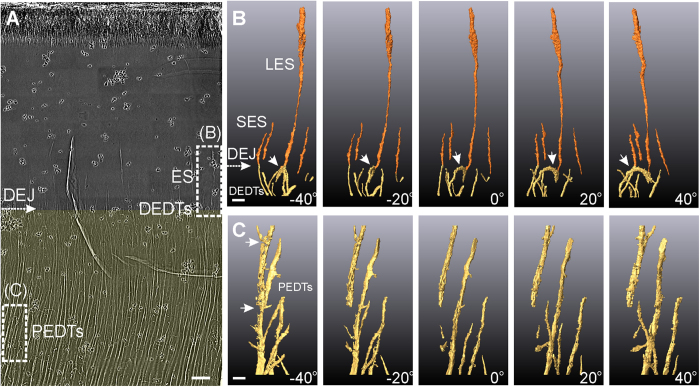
Ultrahigh-resolution internal hollow microstructures of a *Saurolophus* tooth obtained via TXM. (A) 2D X-ray photograph of a sectioned *Saurolophus* tooth. White-dashed rectangles indicate the 3D reconstruction regions shown in (B,C), with the viewing angles normal to incident X-rays at −40°, −20°, 0°, 20°, and 40°. Dentin is colored translucent yellow, and enamel is uncolored. (Scale bar: 10 μm.) (B) Long and short enamel spindles (LES and SES, in orange) and distal ends of the dentinal tubules (DEDTs, in yellow). The bulbous LES and small SES are approximately 30 μm and 10 μm in length, respectively. White arrows indicate a curved dentinal tubule (DT) near the dentinoenamel junction (DEJ). (Scale bar: 2 μm.) (C) Proximal ends of the dentinal tubules (PEDTs), which are approximately 70 μm away from the DEJ. White arrows indicate structural defects in the DTs. (Scale bar: 2 μm.) (The small black dots in (A) are gold particles, which are used for system and image positioning).
